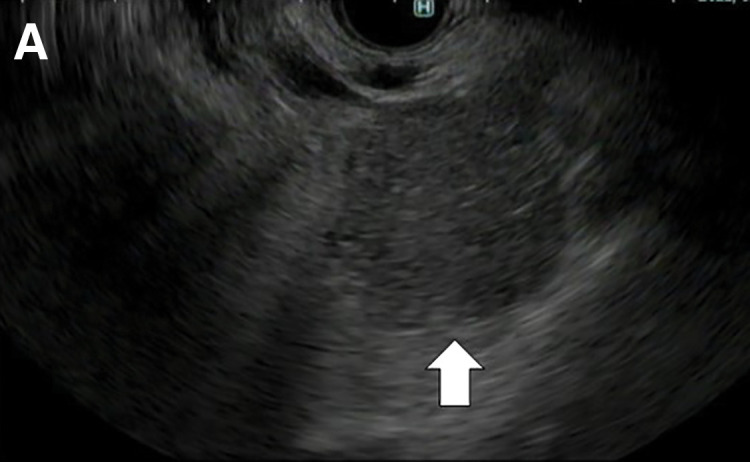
EUS shows a 30-mm hypoechoic mass with irregular margins in the pancreatic head (arrow). EUS, endoscopic ultrasonography
